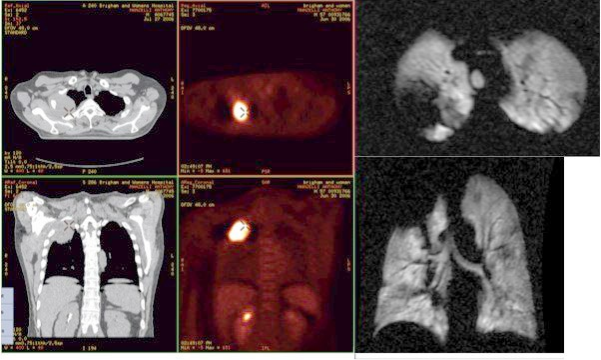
Patient #1 – right upper lobe mass demonstrated on fused PET/CT, with axial (upper) and coronal (lower) slices of Helium MRI.
